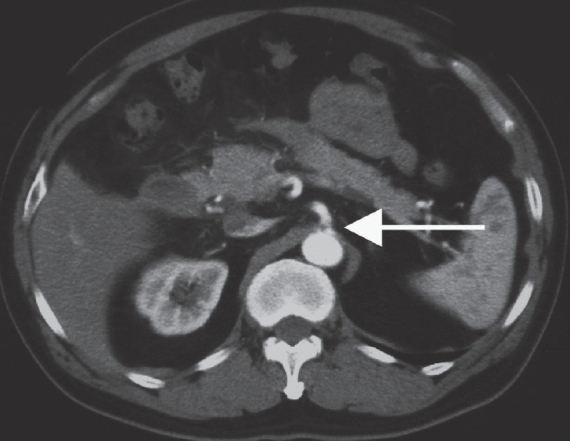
Contrast-enhanced CT scan shows a prominent median arcuate ligament (arrow) compressing the origin of the celiac axis
